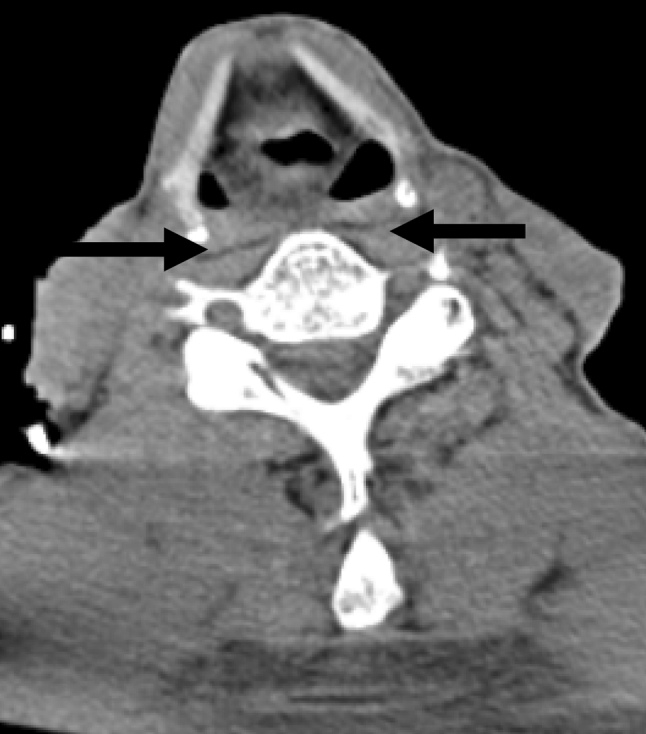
Upper esophageal orifice (*arrows*) is clearly revealed on unenhanced axial CT image
